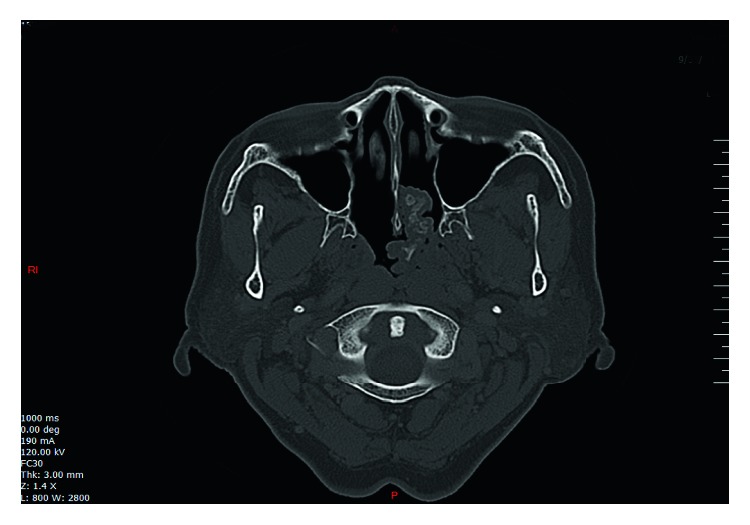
CT brain demonstrating left nasal mass with nasopharyngeal extension.
